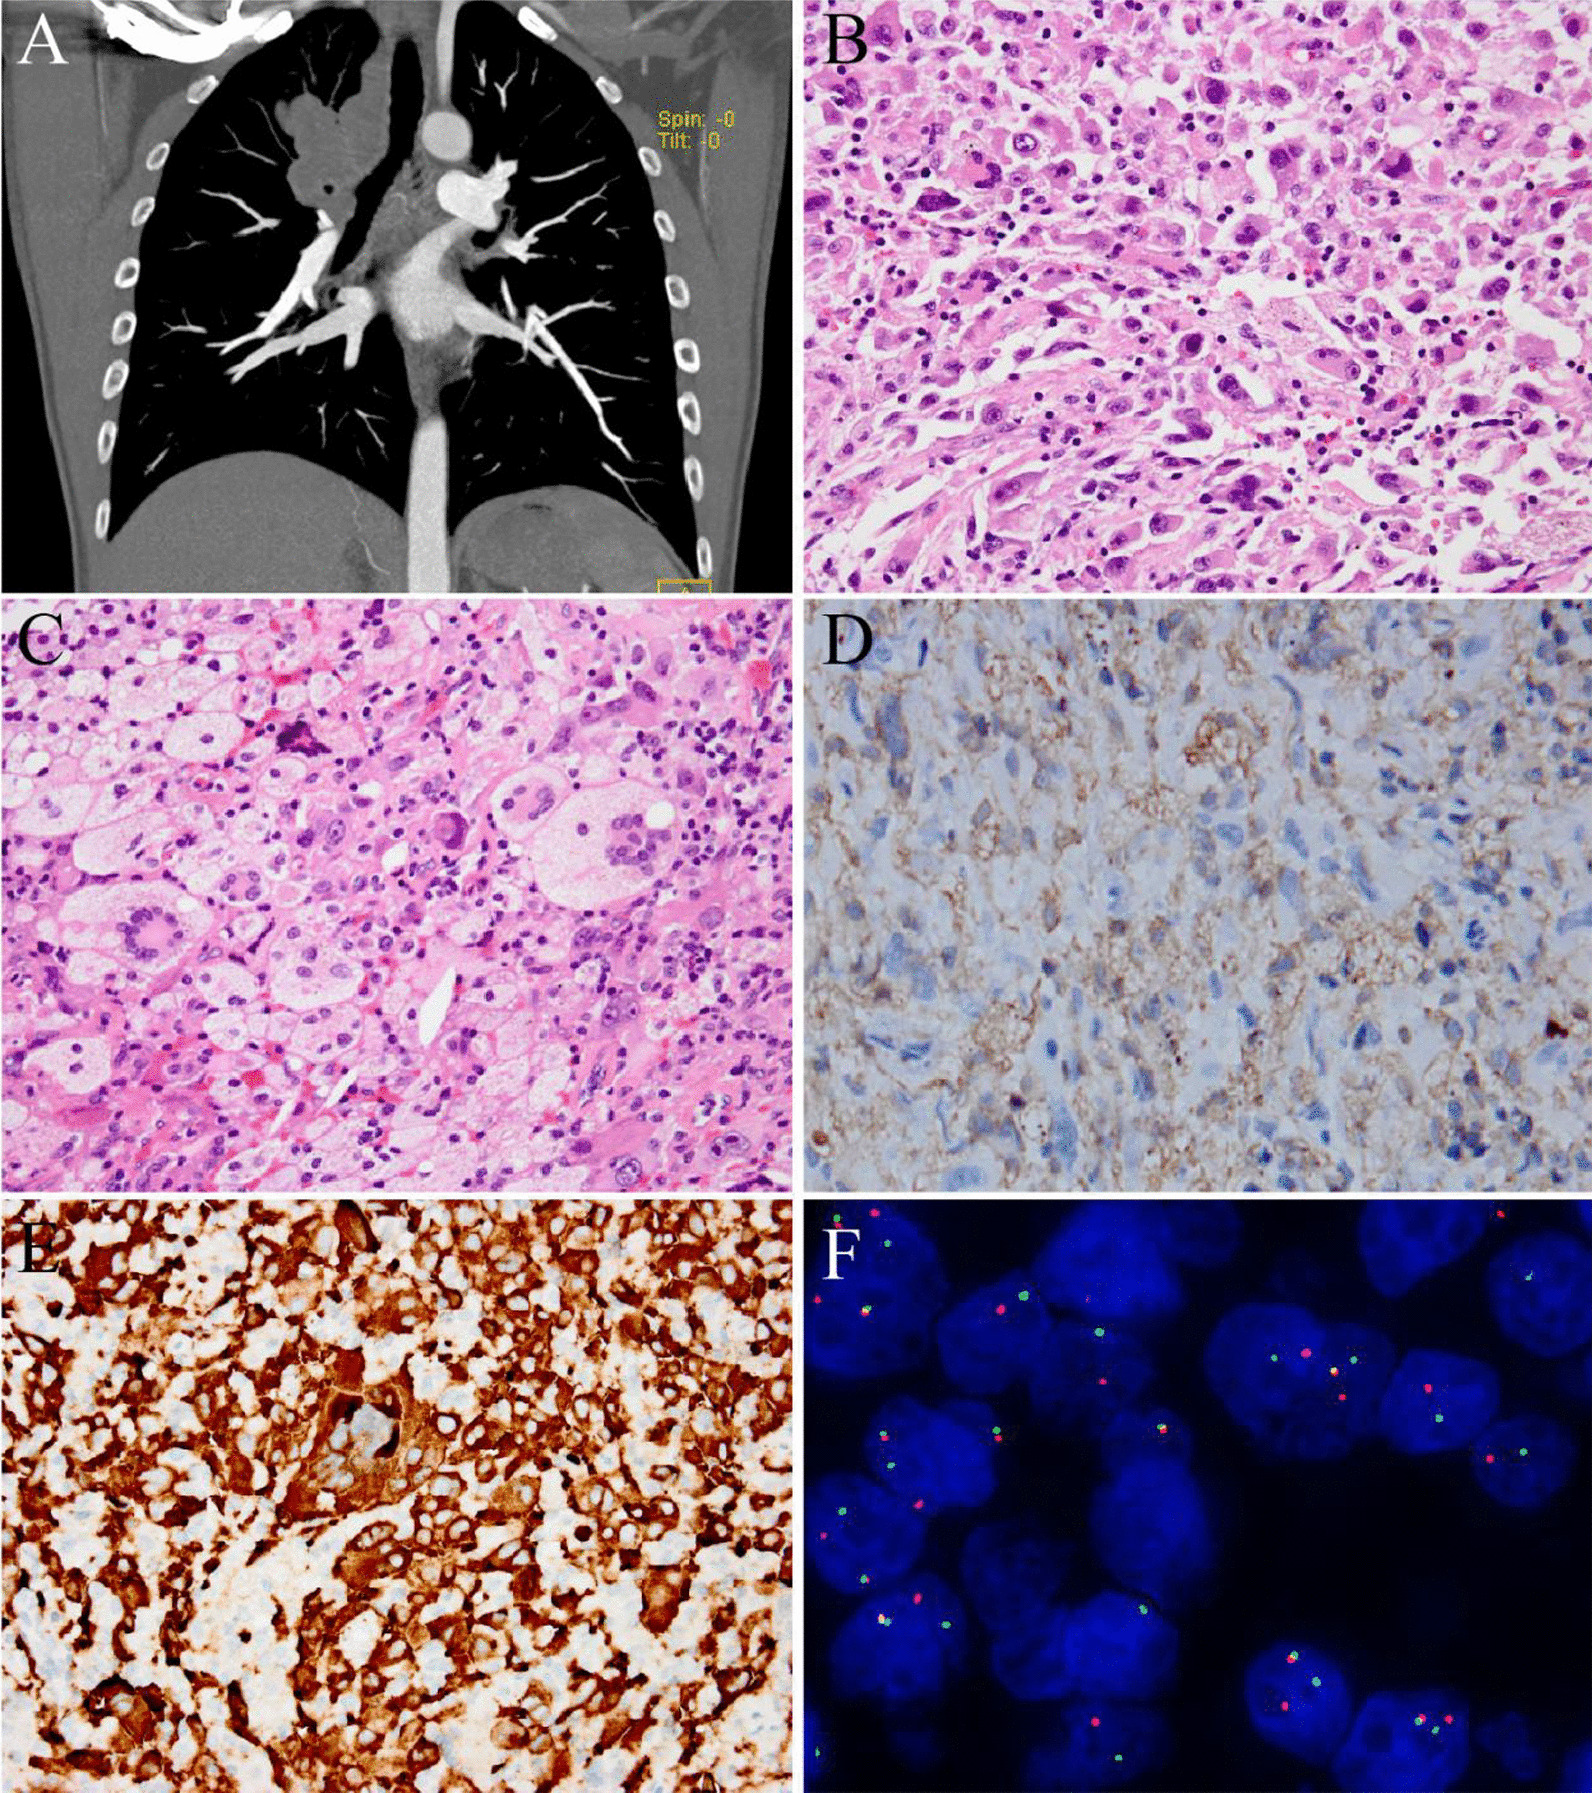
Radiological and pathological features of case 1. A Chest CT showed a large mass in the upper lobe of the right lung, involving the right anterior superior mediastinum, hilar, and hilar lymph nodes. B, C The mediastinal nodule exhibited large polymorphic histiocytes with frequent Touton giant cells. D The tumor cells were positive for CD68. E The tumor cells were positive for ALK1. F ALK-FISH (a break-apart probe) was positive. (Original magnification × 200 [B, C, D, E]; × 1000 [F])
